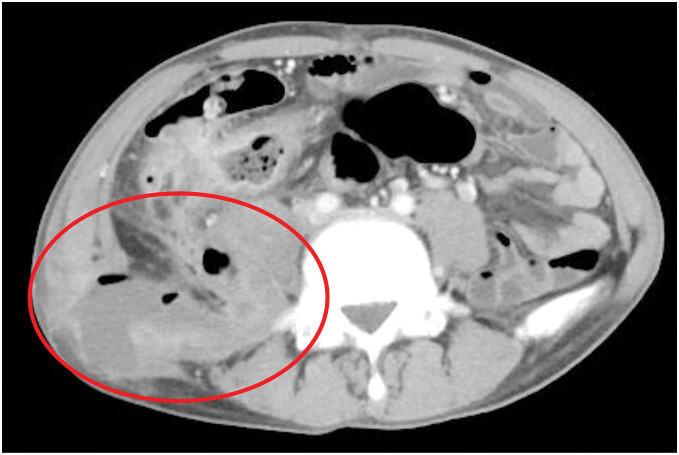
CT findings at admission. The image shows an abscess extending from near the right ilium to the iliopsoas muscle, with recurrence of the previous right retroperitoneal abscess. The circled area indicates the abscess cavity.
